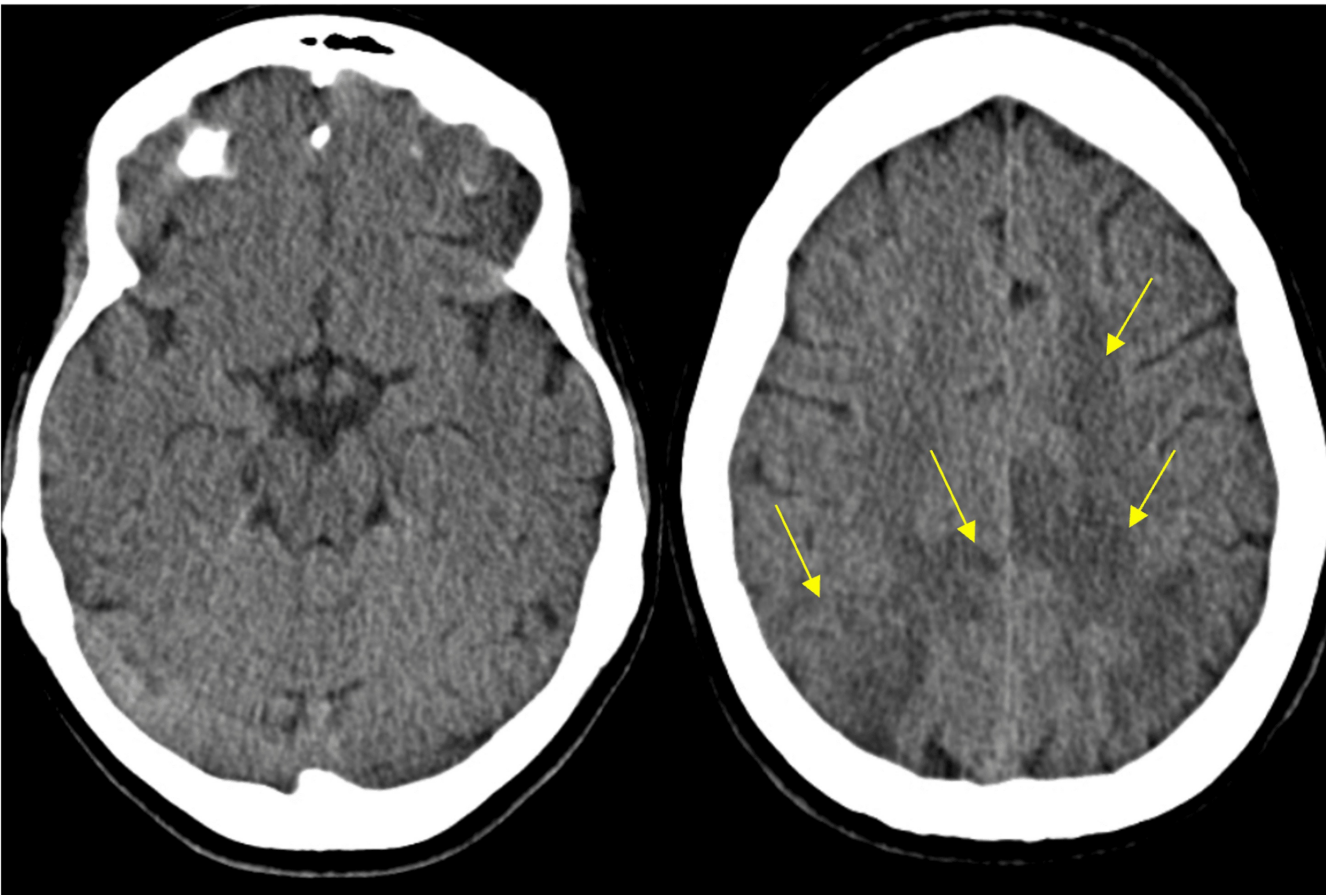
Emergency department cranial CT Hypodense areas involving the white matter bilaterally in high fronto-parietal subcortical planes, and subcortical and cortico-subcortical areas of the bilateral parieto-occipital region (yellow arrows), suggestive of vasogenic edema CT: computed tomography
